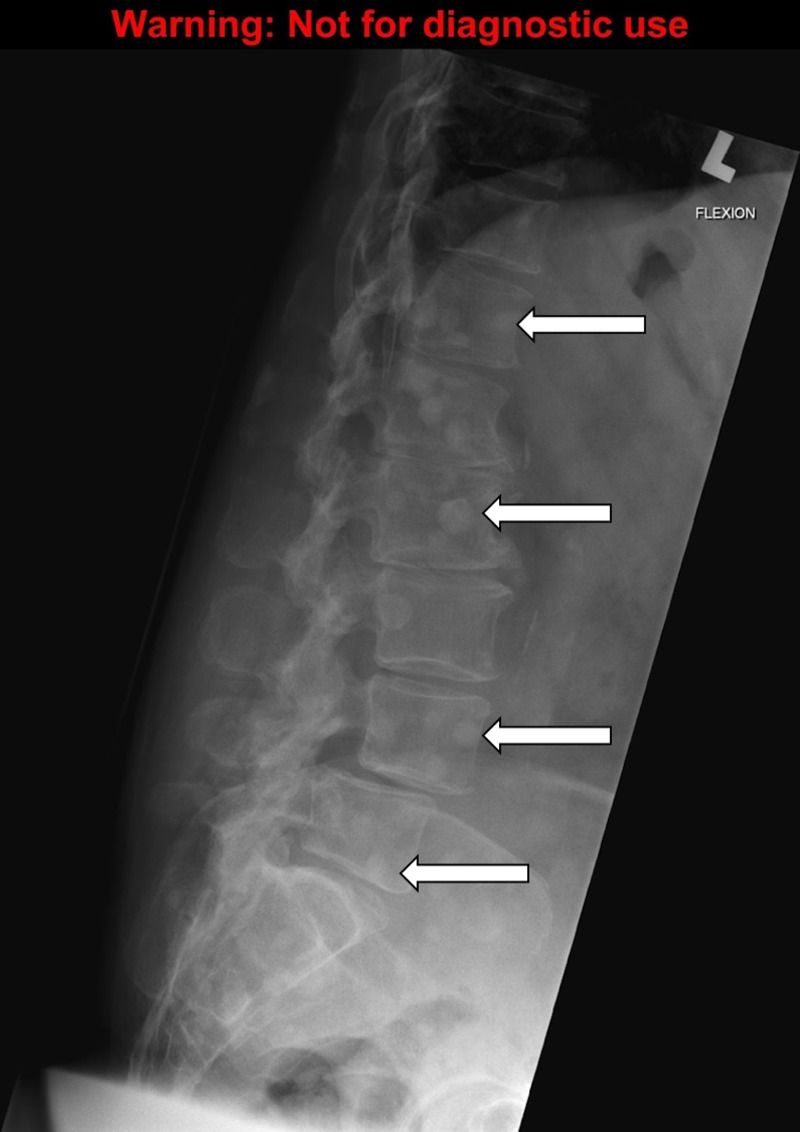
The lumbar X-ray demonstrating bone metastasis. Source: A Dixit
